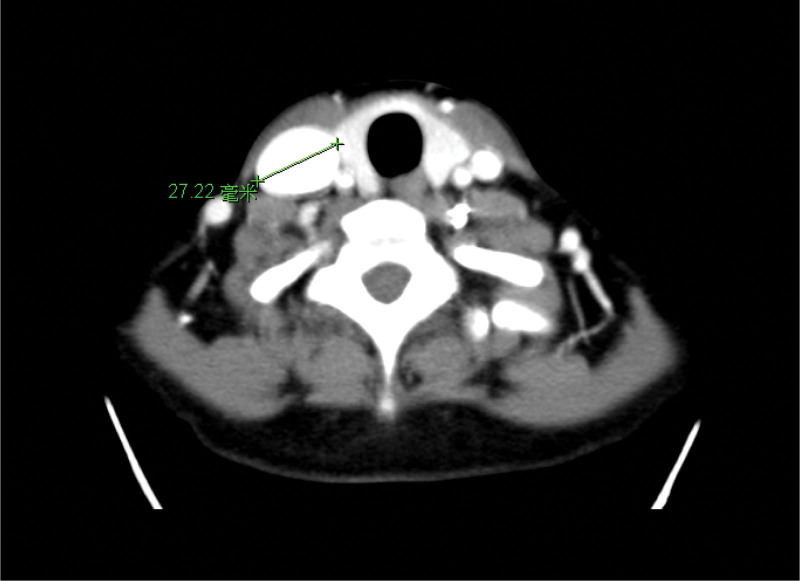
Axial view of head and neck CT showed a right fusiform internal jugular vein aneurysm, 27.22 mm in maximum diameter. CT = computed tomography.
